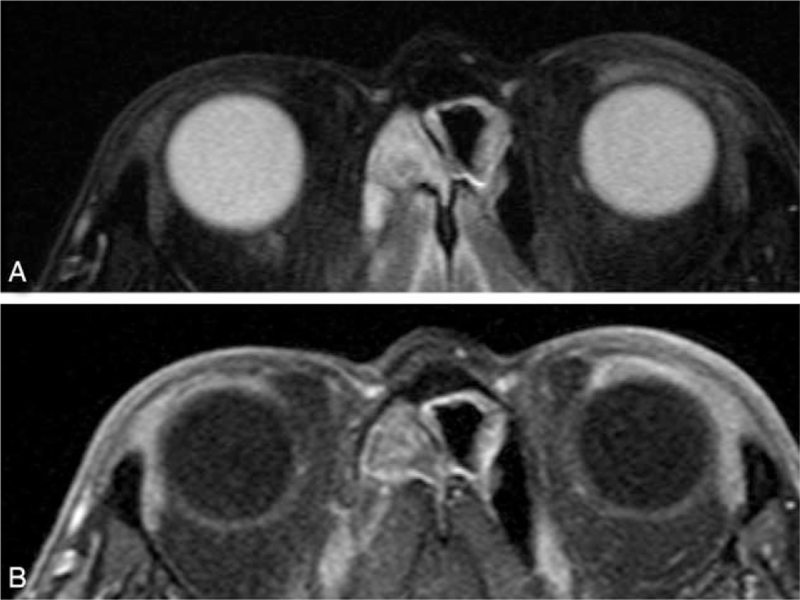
A magnetic resonance image showing the mass on the temporal side of the left eye with a contrast effect. (A) T2 weighted imaging. (B) Contrast-enhanced T1-weighted imaging.
